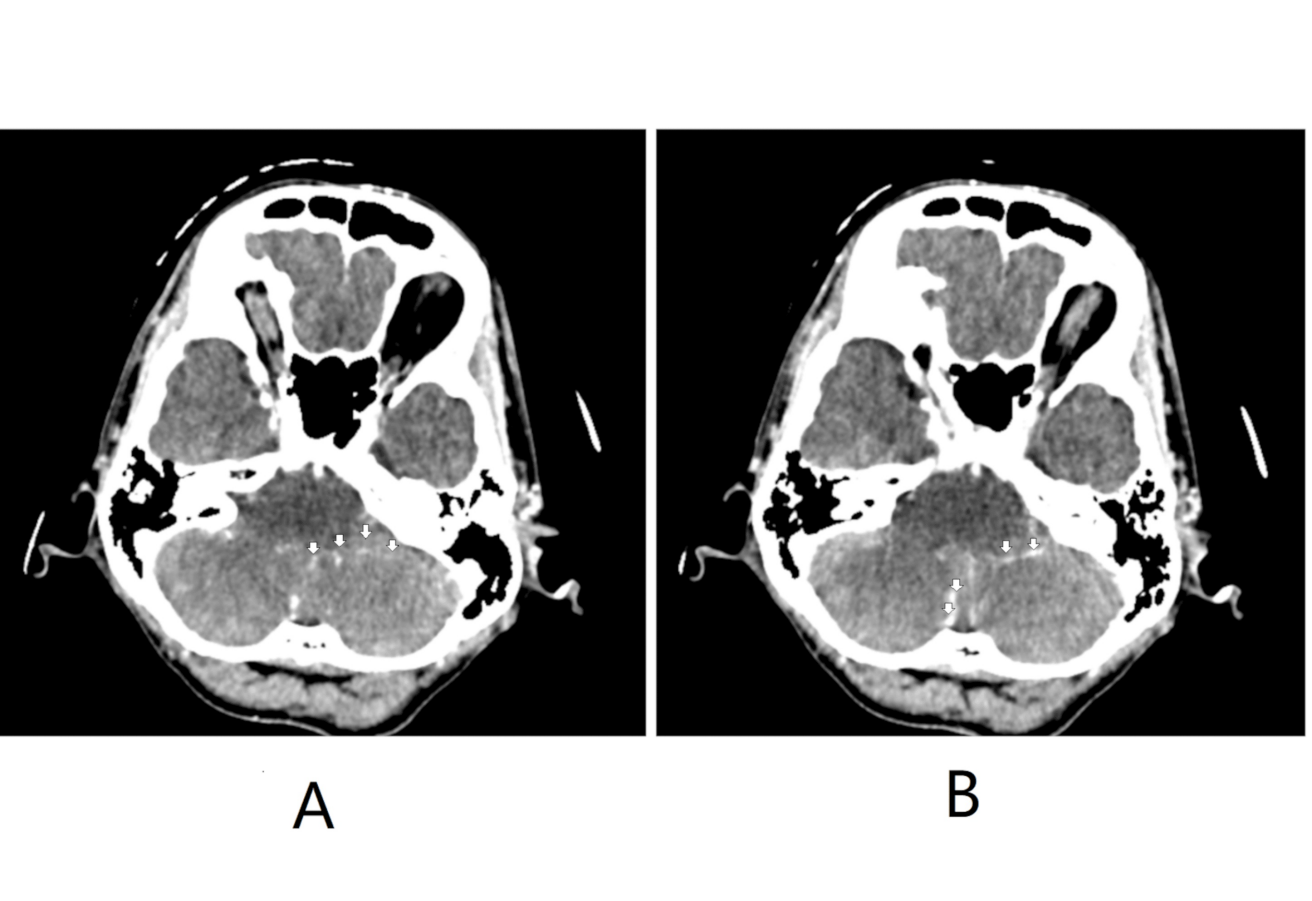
Axial contrasted CT of the brain taken at the level of the posterior fossa/cerebellum A: Multiple enhancing foci are seen within the cerebellar parenchyma (marked by arrows). The lesion adjacent to the aqueduct of Sylvius was 1.2x1.6 cm, causing compression and dilatation of the bilateral lateral and third ventricles. B: Leptomeningeal enhancement is seen at the midline anterior lobe of the lower cerebellum.
